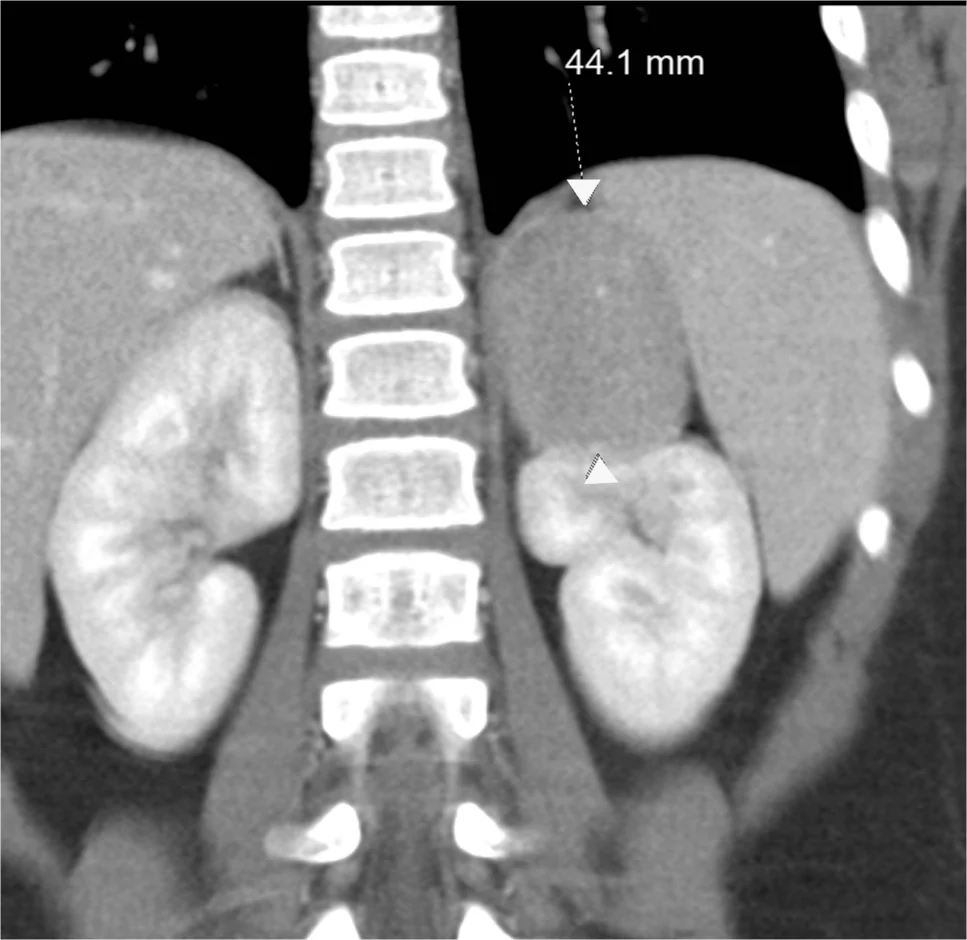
Virilizing adrenal cortical adenoma in a 4-year-old female who presented with pubic hair. Laboratory studies were notable for mildly elevated androstenolone sulfate at 89 mcg/dL (normal < 34) and elevated testosterone of 134 ng/dL (normal < 9). Coronal image reconstruction from a contrast-enhanced abdominal CT exam in portal venous phase shows a homogeneous, well-demarcated left suprarenal mass (*arrowheads*) maximally measuring 4.4 cm in craniocaudal height, consistent with functional adenoma in this clinical setting
